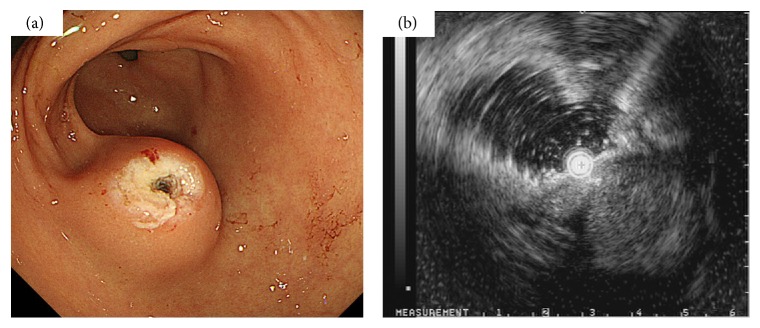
Upper gastrointestinal endoscopy and endoscopic ultrasonography findings. The image shows a well-circumscribed elevated mass, measuring 30 × 30 mm, with normal overlying mucosa in the anterior wall of the gastric antrum (a). The image shows a well-demarcated mass in the third and fourth layers of the gastric wall with a hypoechoic pattern (b).
